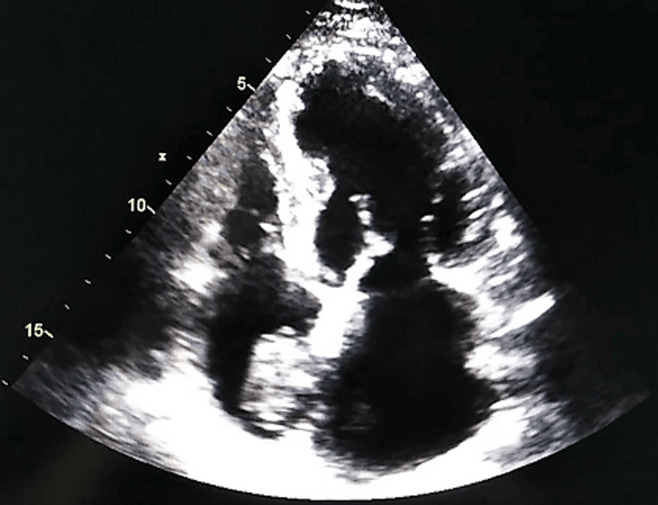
TTE apical four chambers view showing a thrombus in the right atrium, attached to the inter-atrial septum
